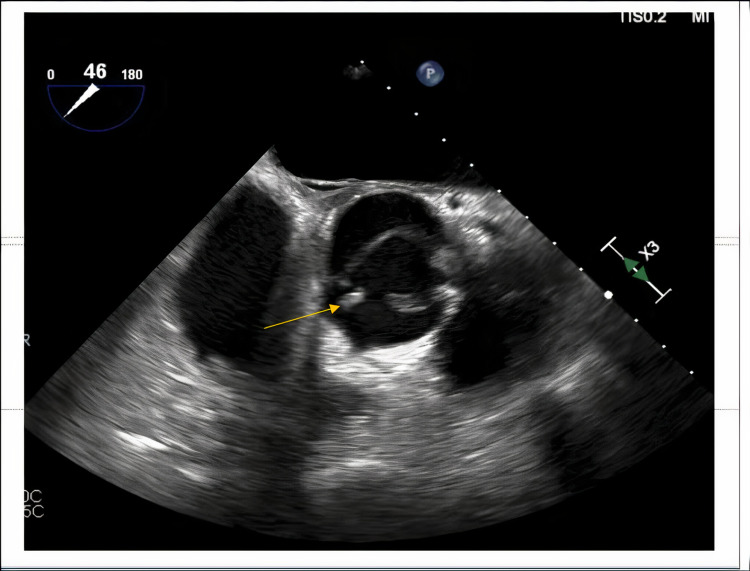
Transesophageal echocardiogram showing linear echo density measuring 1.9 cm on the aortic aspect of the aortic valve concerning for vegetation (yellow arrow).
